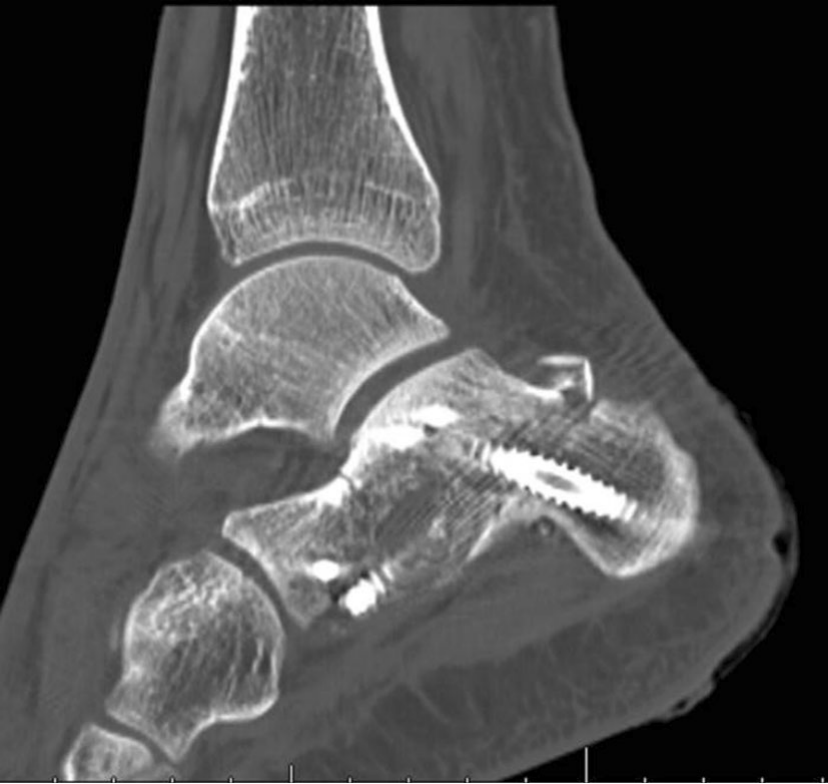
Post-operative sagittal CT views
